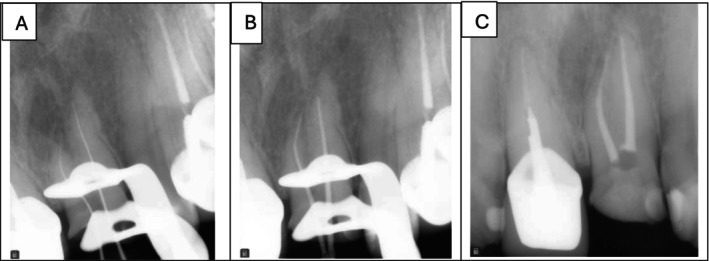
Endodontic procedural radiographs. (A) Working length radiograph. (B) Master cone fit radiograph. (C) Post‐obturation radiograph.
